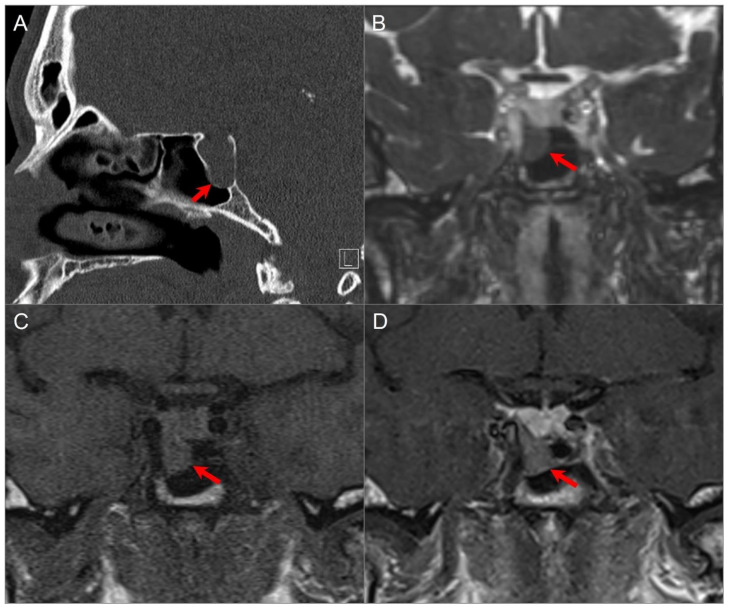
Exophytic/ectopic-intrasphenoidal pituitary adenoma. (A) Sagittal CT image shows erosion of the sellar floor with a soft tissue lesion extending into the clivus and posterior margin of the sphenoid sinus (red arrow). (B) Coronal postcontrast CISS and (C) noncontrast T1W images reveal a hypointense lesion abutting the inferior margin of the right pituitary lobe and medial wall of the petrocavernous ICA. (D) Coronal postcontrast T1-weighted image demonstrates normal enhancement of the pituitary gland and hypoenhancement of the exophytic adenoma (red arrow).
